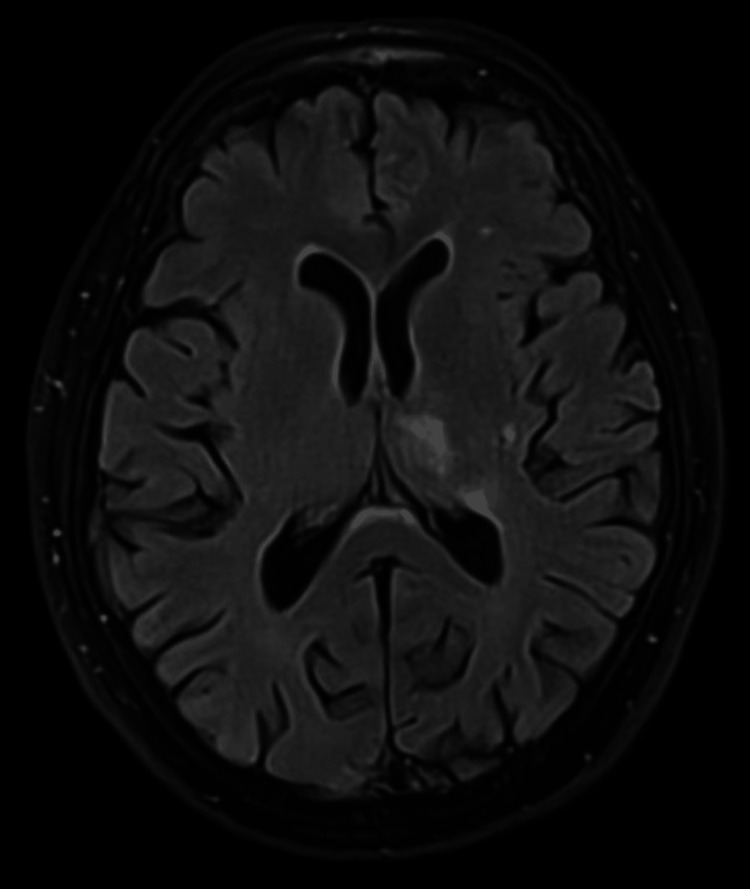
Magnetic resonance imaging showing acute infarct involving the posterior limb of the left internal capsule, extending into the medial temporal lobe and caudate nucleus
